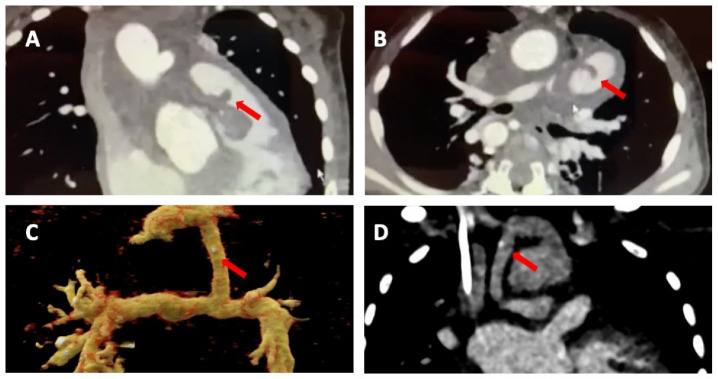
Cardiac computed tomography infective endocarditis visualisation. The images show right-ventricular pulmonary artery (RV-PA) conduit involvement (panels (A,B)) and systemic to pulmonary shunt involvement with a calcified lesion (panel (C,D)). The arrows indicate the vegetations.
